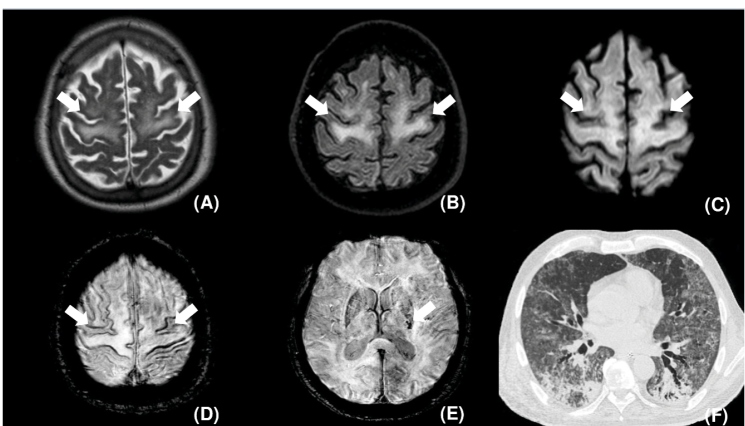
Axial T2 (A), FLAIR (B), and diffusion (C) weighted images demonstrating symmetric diffuse hyperintensity (arrows) and mild restricted diffusion (arrows) involving the subcortical white matter in the precentral gyrus and the posterior portions of the superior and middle frontal gyrus. Axial susceptibility-weighted images, (D) and (E), depict punctate microhemorrhagic foci on the cortical surface of the precentral gyrus, middle frontal gyrus, and superior frontal gyrus (arrows in D) and in the posterior limb of the internal capsule (arrow in E). Chest CT, pulmonary window, axial: diffuse areas of ground-glass opacities and consolidations (F).
